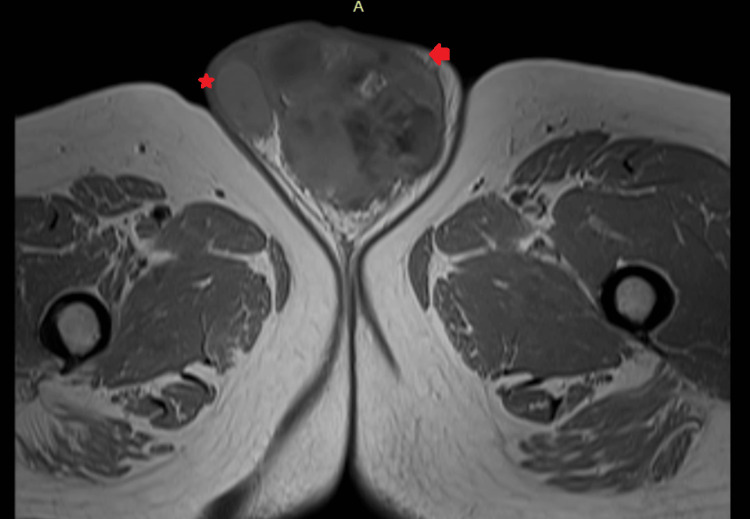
MRI T1 sequence showing "*" (asterisk symbol) as the unaffected testis on the right side of the patient, and "→" (arrow head symbol) as the affected testis on the left side of the patient.
